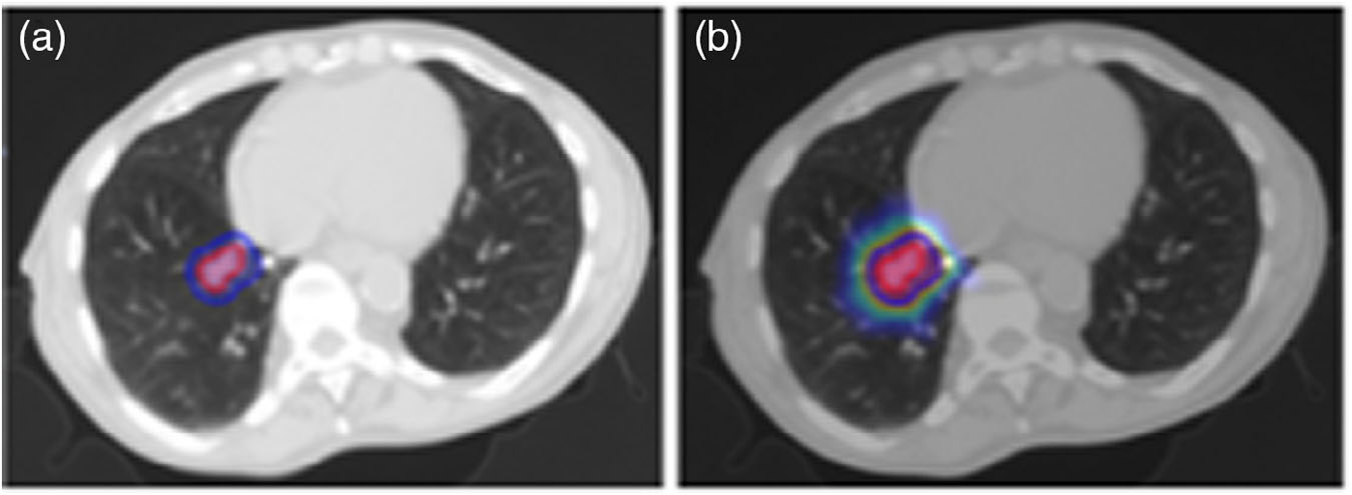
A 66‐year‐old male with Stage IA2 (cT1bN0Mx) adenocarcinoma in the right lower lobe of the lung. Images procured from our institution. (a) Axial view of the planning CT displaying CTV and PTV contours. (b) Axial view of the planning CT with CTV and PTV contours and a dose color wash, with a dose window level of 20 to 50 Gy.
